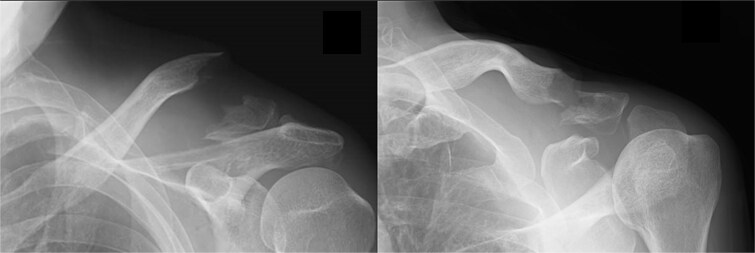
Radiographs captured immediately after injury show a fracture of the left distal clavicle with displacement.
